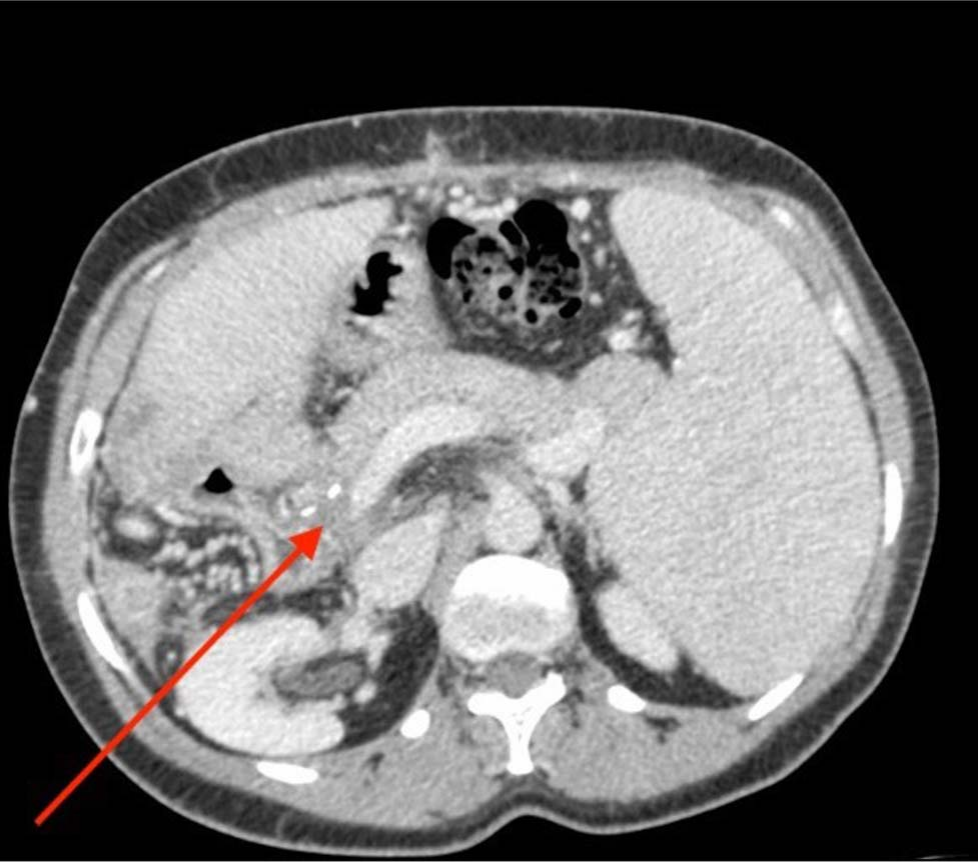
Postoperative computed tomography scan (2005) demonstrating portal vein thrombosis.
